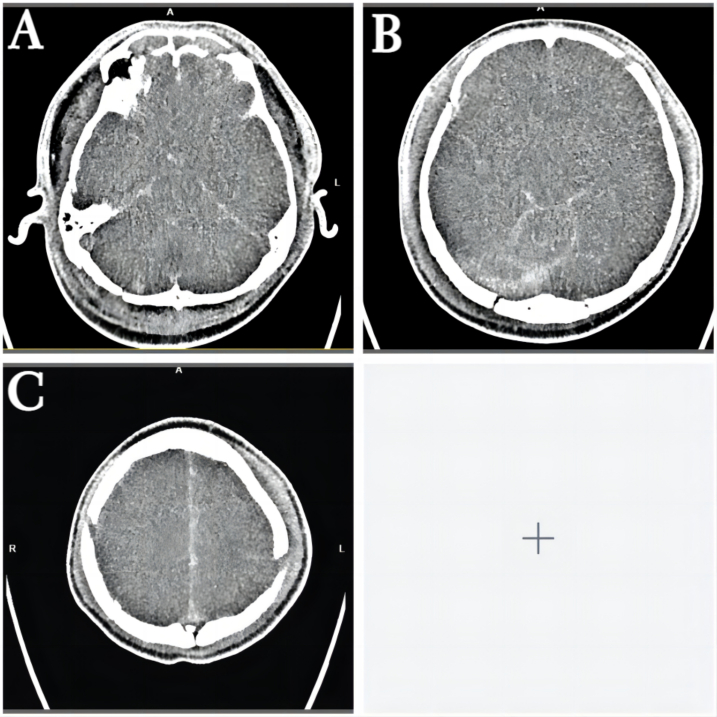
(A and B and C)Imaging of the patient's follow-up head CT on the 5th day of admission.
